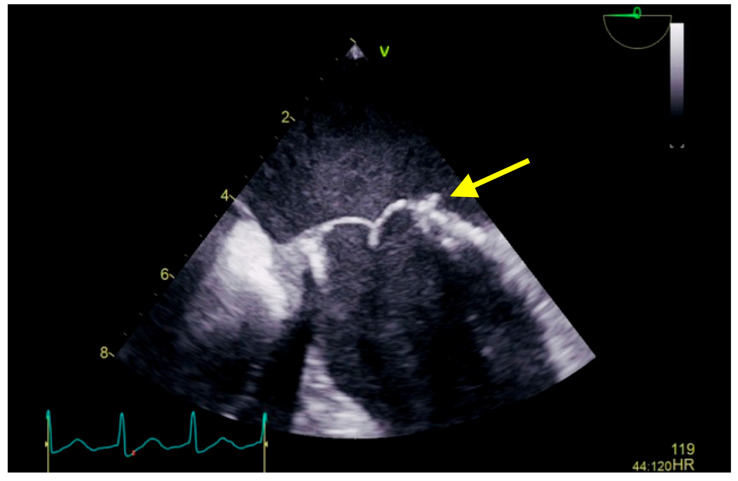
Transesophageal echocardiography showing a biological prosthesis in the mitral position, yellow arrow showing a reduced dimensions of the vegetation compared to the previous examination. A 6 months follow-up revealed stationary aspect.
